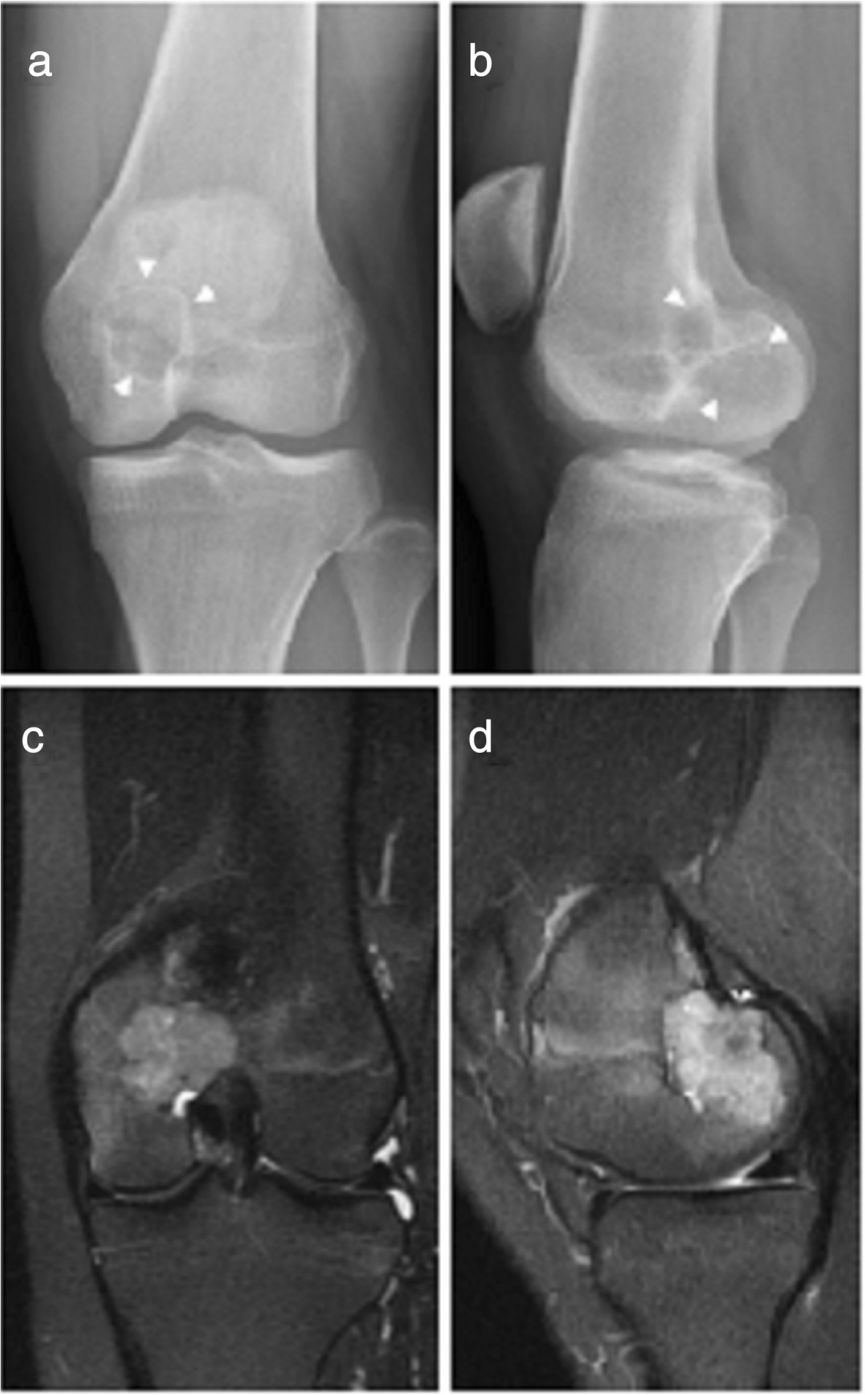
Lesion in the distal epiphysis of the left femur showed marginal sclerosis on radiographs (a,b:arrowheads) and bone edema in magnetic resonance T1-weighted Gd-enhanced images (c,d).
